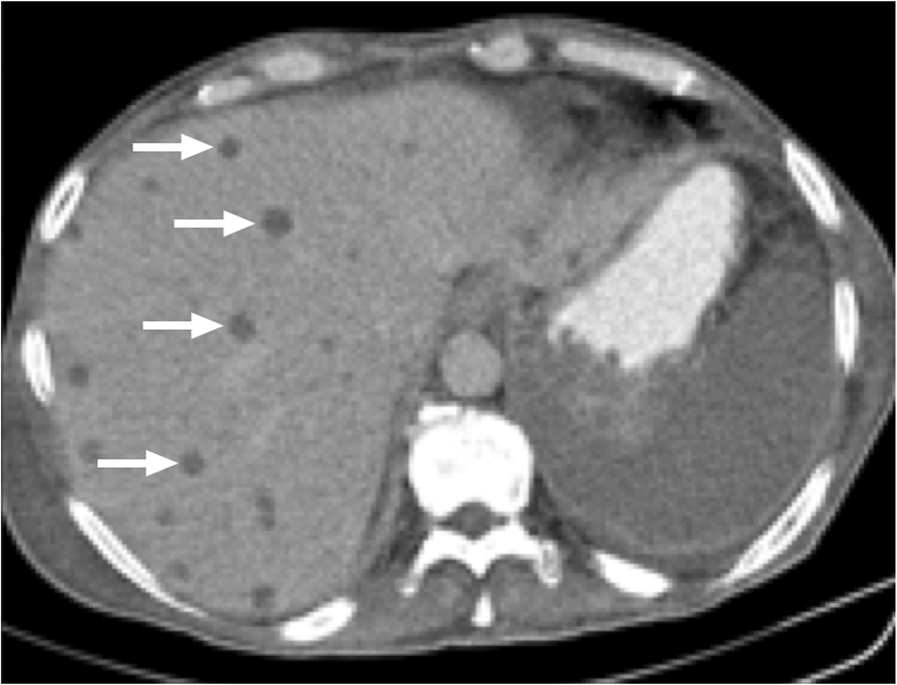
A 61-year-old male with recently diagnosed AML undergoing chemotherapy now presenting with neutropenic fever. Axial plane postcontrast CT image demonstrates multiple well-defined hypodense liver lesions (arrows). Clinical and laboratory findings were consistent with candida infection. The findings almost completely resolved after antifungal treatment (not shown)
